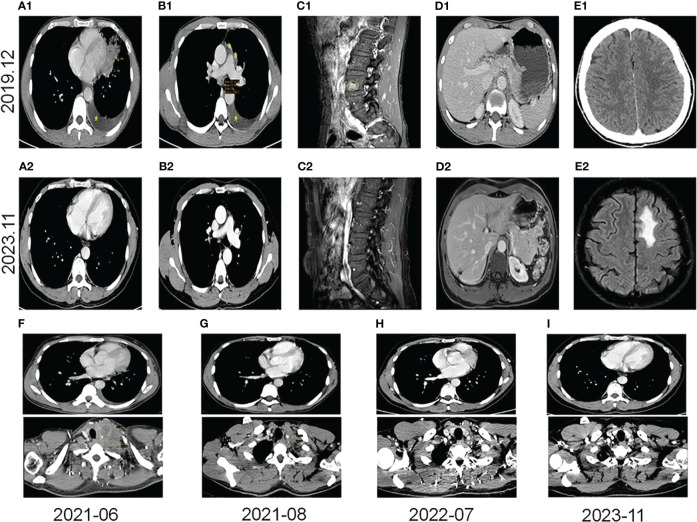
Images from pre-targeted therapy (December 2019). (A1) Soft tissue mass near the heart margin of the lower lingual segment of the left upper lobe (x) and a small effusion of the left thoracic cavity (*) on the CT scan. (B1) Lymph node metastases (○), pleural metastases (#), and a small effusion of the left thoracic cavity (*) on the CT scan. (C1) Spinal metastasis on the MRI scan (○). (D1) A CT scan of liver. (E1) A CT scan of the brain. Images from post-immunotherapy (November 2023). (A2) No mass at the left superior lobe lingual segment on the CT scan. (B2) No nodules of mediastinum and hilum on the CT scan. (C2) Inactive of the spinal metastasis on the MRI scan. (D2) A change after ablation of the liver S7 segment on the MRI scan. (E2) Radionecrotic inflammatory response of the left frontal lobe on the MRI scan. Changes in the left lung mass and the left supraclavicular fossa lymph nodes on the CT scans during immunotherapy (F–I). (F) The left supraclavicular fossa mass (52x69mm) and the left lung mass before immunotherapy; (G) The left supraclavicular fossa mass (19x20mm) and on the left lung mass after 2 months of immunotherapy. (H) Without any masses after 13 months of immunotherapy. (I) Without any masses after 29 months of immunotherapy.
